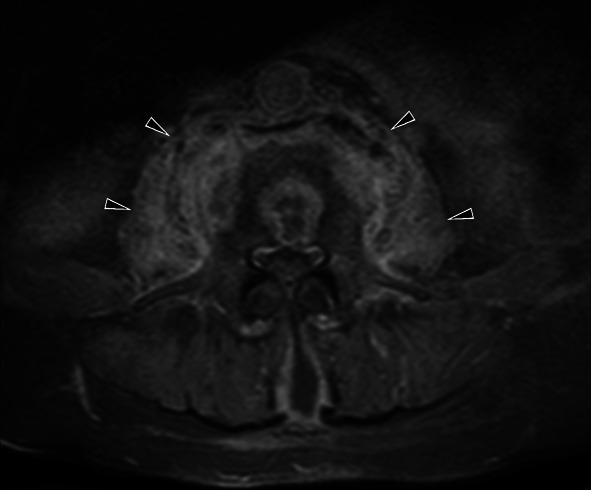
Contrast-enhanced T1 weighted axial MR image of a 52-year-old male appears an inflammatory mass lesion within the adjacent soft tissues to the body of the fourth lumbar vertebra be caused by a phlegmon and abscess
